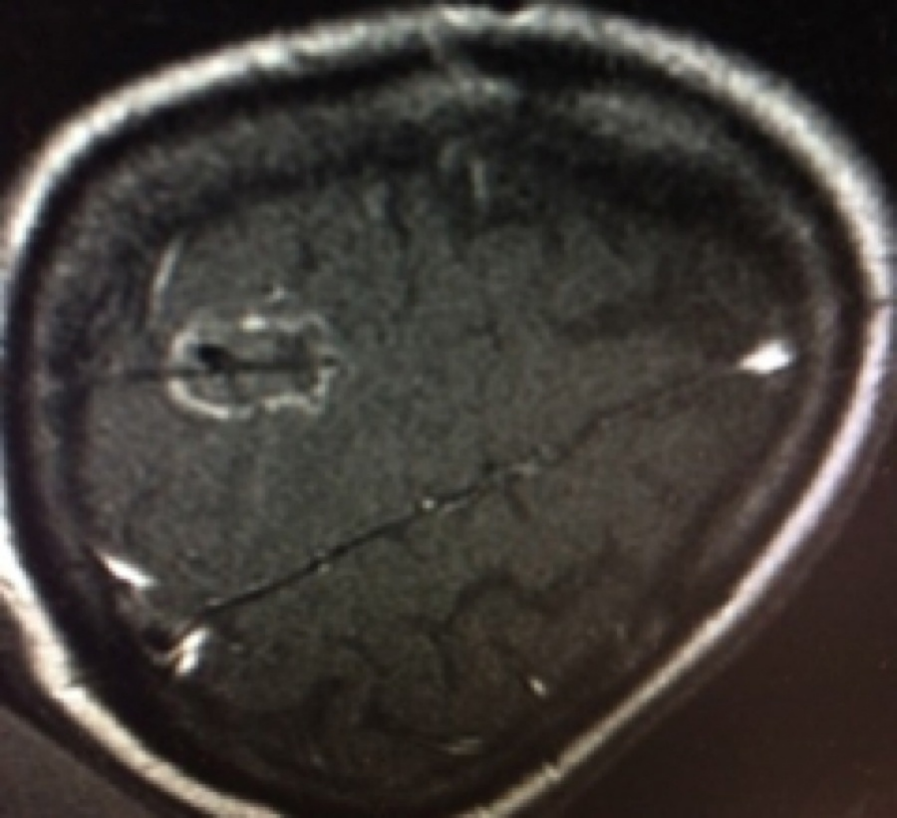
Six-Month Follow-up Scan Postoperative axial T1 post-gadolinium MRI scan at the six-month follow-up demonstrates evidence of the original tract into the lesion, with no new areas of enhancement concerning for tumor recurrence.
